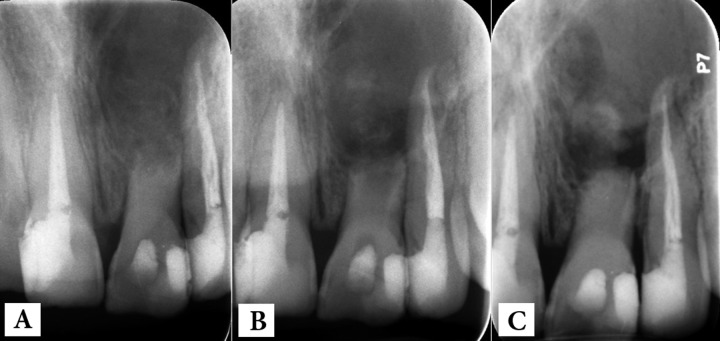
Post treatment radiographs: *A) *immediately after CH therapy;* B) *one week later (CH washing out) and* C) *CH replacement
